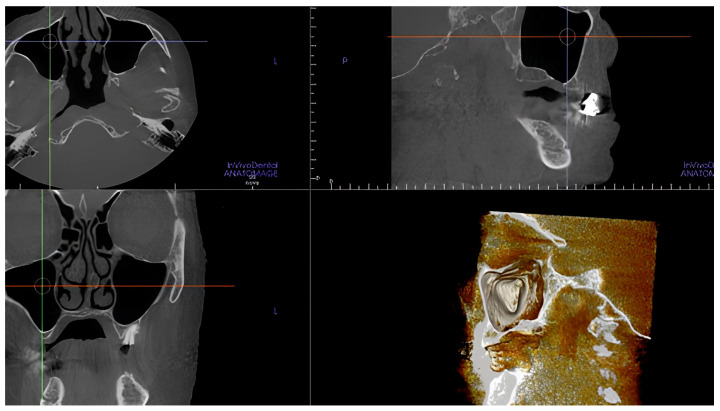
CBCT scans and volume rendering using in vivo dental Anatomage showing the artery was not detectable.
